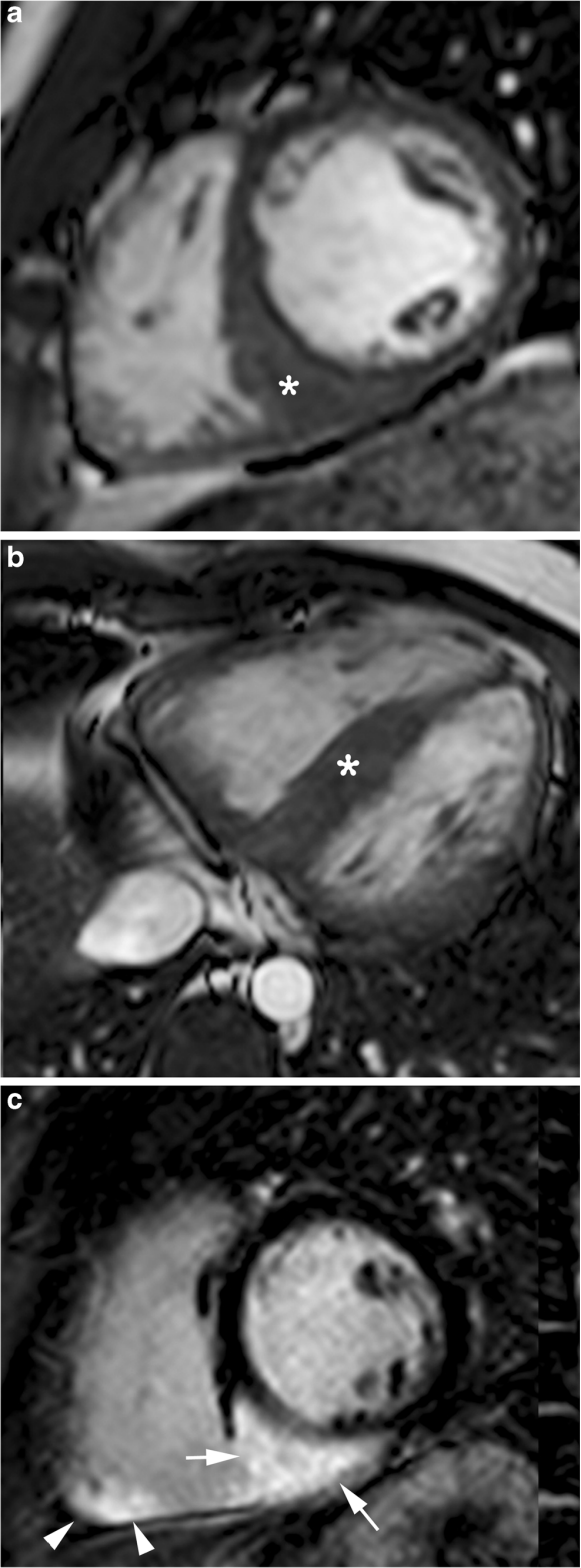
A 29-year-old man with pulmonary sarcoidosis who had recurrent ventricular tachycardia and negative coronary angiography findings. a Short-axis and (b) axial cine SSFP MR images at end-diastole show basal septal myocardial thickening (*asterisks*). c Short-axis post contrast T1-weighted inversion recovery gradient echo image shows subepicardial LGE (*arrows*) in the inferoseptal and inferior walls of the left ventricle, as well as subendocardial LGE (*arrowheads*) in the inferior wall of the right ventricle. Functional assessment (not shown) demonstrated 35% left ventricular ejection fraction
